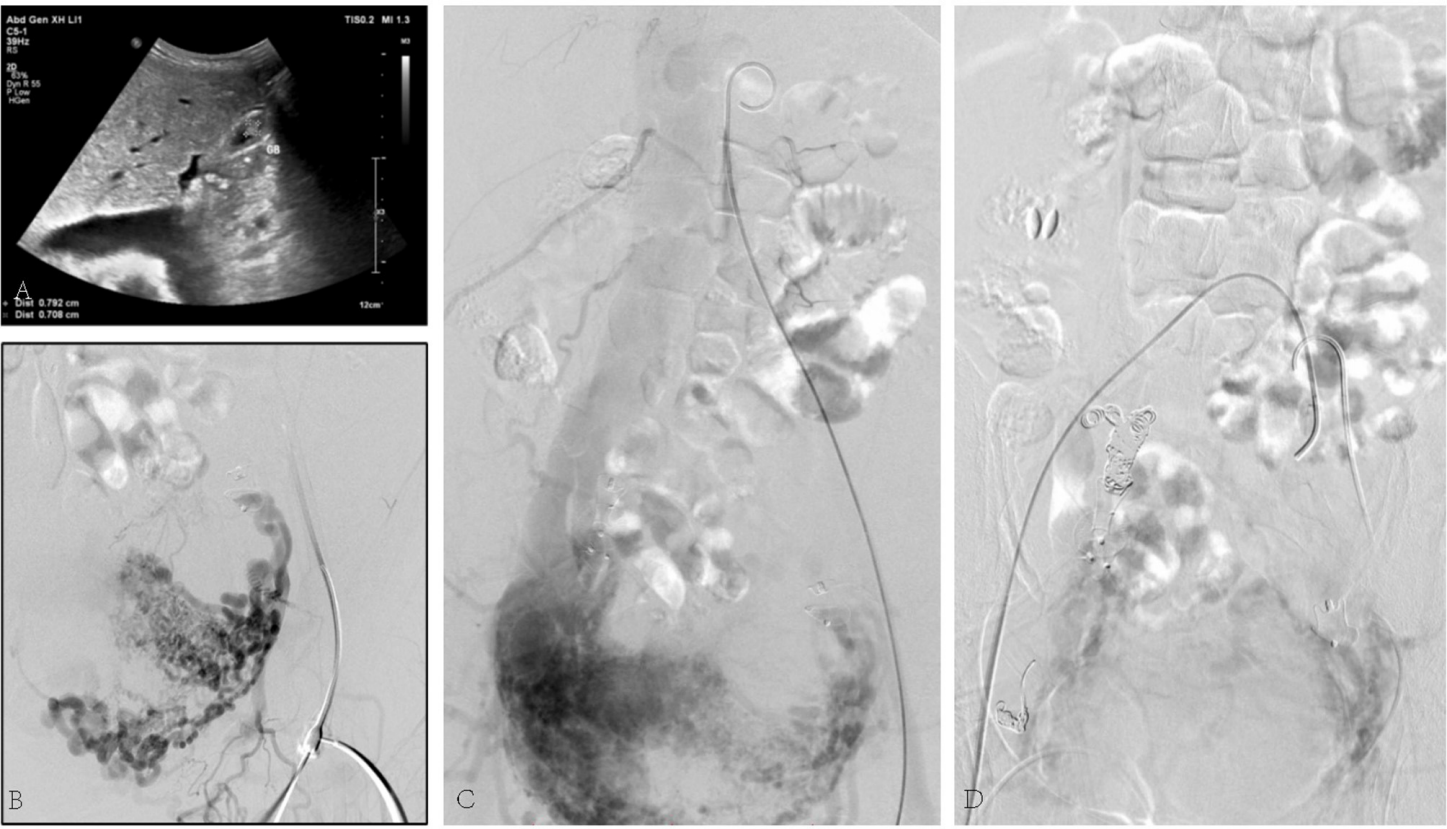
(A) Ultrasound revealed congested liver. (B) Arteriovenous malformation. (C) Shunt flow entering the vein. (D) Abnormal flow stopped by the placement of embolization coils.
